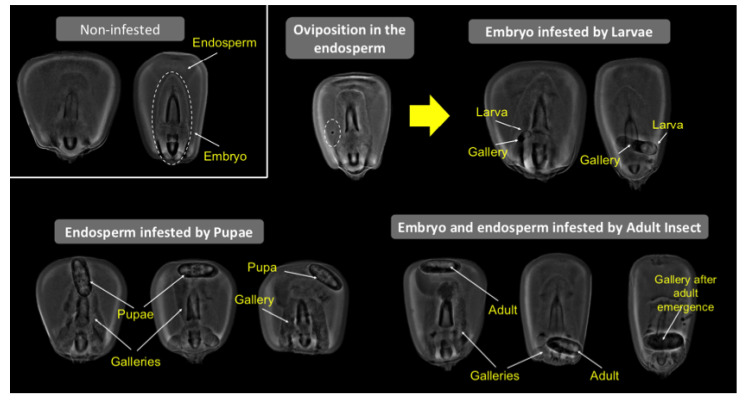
X-ray imagens processed by peripheral equalization and calcification emphasis algorithms for classes of non-infested maize grains and grains infested by *Sitophilus zeamais* showing damages (“galleries”) in different parts of the grain.
